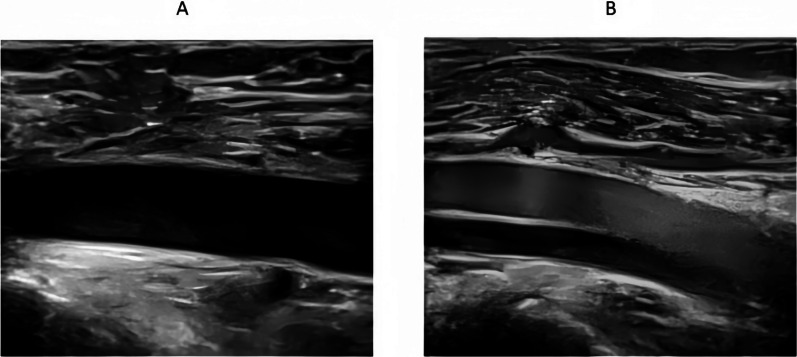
Classification of the “blizzard” sign on Doppler ultrasound. The marginal type “blizzard” sign is characterized by hyperechoic swirling echoes localized along the vessel wall. The diffuse type presents as dense, echogenic signals distributed throughout the venous lumen
